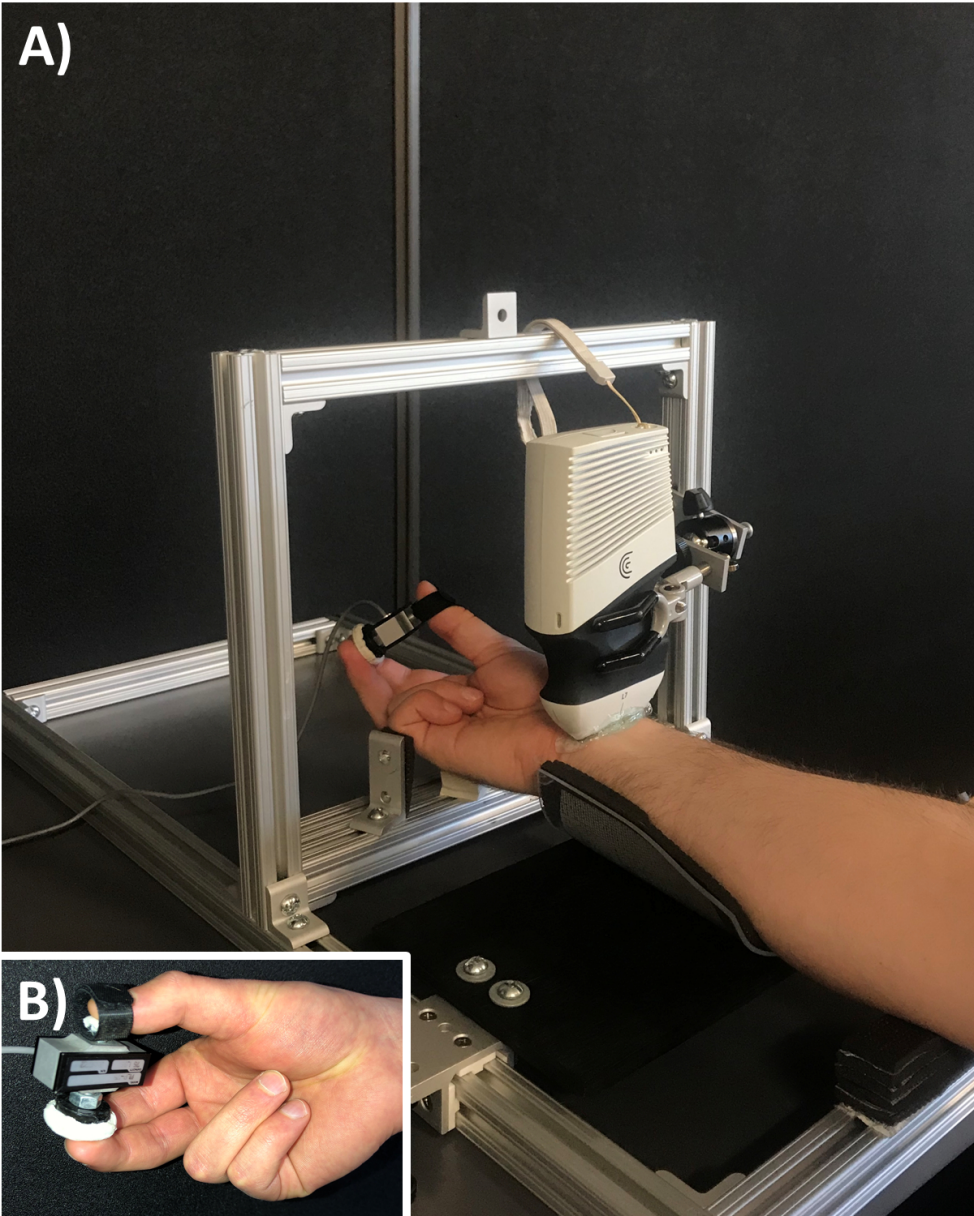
Experimental setup. (A) The custom apparatus was adjustable to support each participant’s anthropometry. The apparatus included supports for the forearm and wrist while a clamp fixed the ultrasound transducer. (B) The load cell consisted of pushbuttons on either side to perform a chuck grip.
